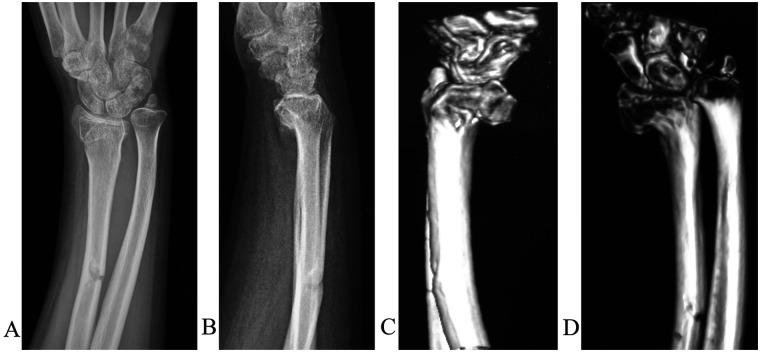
A 36-year-old female patient suffered from depressed distal radial malunion (initial type B3) because of a fracture that occurred 4 months before hospital admission. (A) A posteroanterior (PA) X-ray. (B) A lateral X-ray. (C) A radial view of a three-dimensional CT scan. (D) An anteroulnar view.
